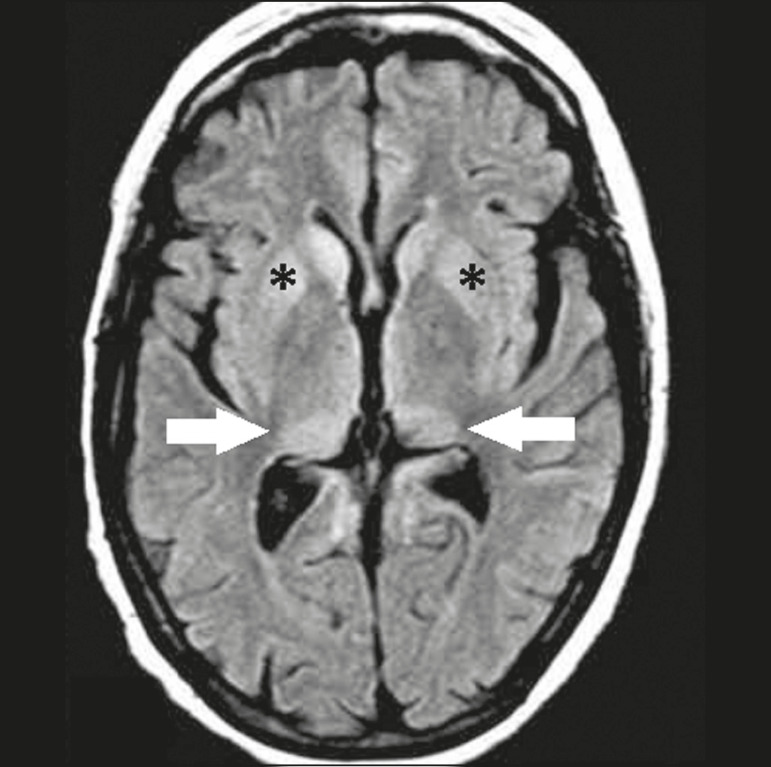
Creutzfeldt–Jakob disease in a patient with rapidly progressive cognitive decline. Axial FLAIR MRI sequence showing bilateral areas of hyperintensity in the thalamus (arrows), as well as in the caudate and lentiform nuclei (asterisks).
